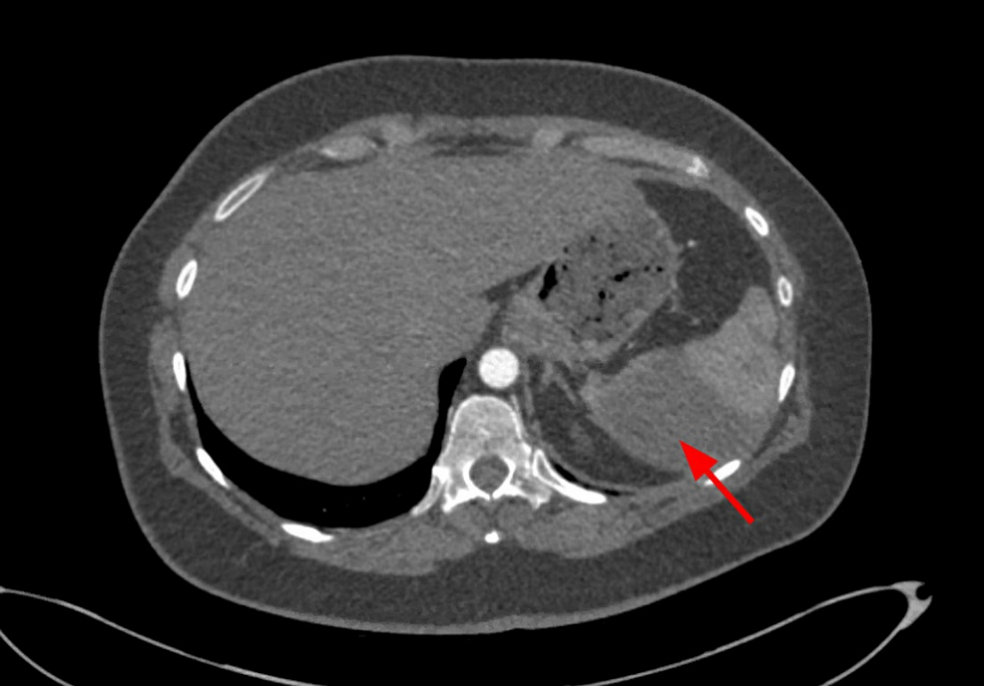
CTA abdomen and pelvis on POD#30 showing the infarct involving the spleen (red arrow) without any obvious abscess. CTA, computed tomography angiography; POD, post-operative day
